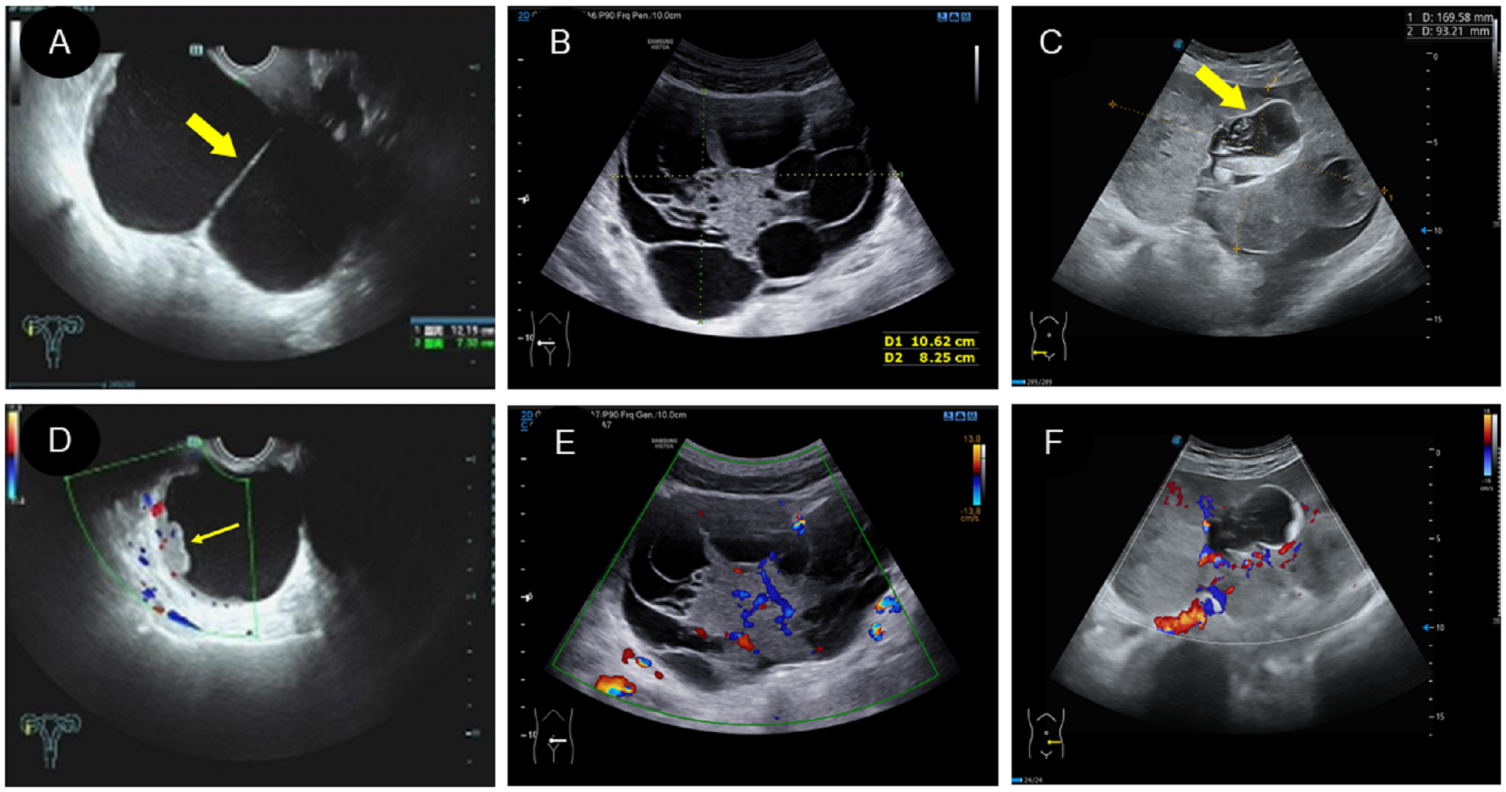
Two dimensional and color Doppler ultrasound findings of different types of yolk sac tumors. (A, D). Cystic yolk sac tumor is a large cyst with regular morphology and clear boundary. The cyst is filled with weak echoes, with septation (thick arrow) and mural papillary nodules (thin arrow) visible on the wall. Color Doppler flow imaging (CDFI) showed punctate blood flow signals in the cystic wall and nodules; (B, E). Cystic solid mixed yolk sac tumor also showed regular morphology and a clear boundary. The solid part showed low echoes, while the cystic part displayed large separation of different sizes and uneven thickness, separating the cystic area into many small chambers. CDFI revealed rich blood flow signals in the solid part and separation; (C, F). Solid yolk sac tumor appears as a large solid mass with a more irregular morphology but a clear boundary. It displayed moderate echogenicity, less uniformity, and partially visible small cystic area (thick arrow), CDFI showed rich internal flow signals.
